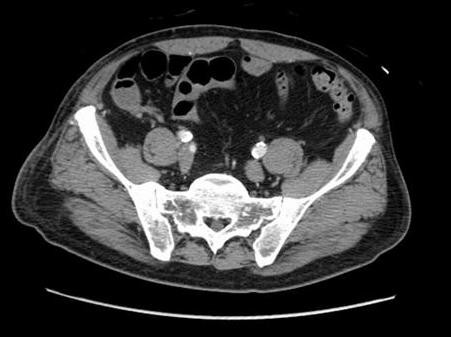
Abdominal CT scan. An angio CT scan showed calcic atheromatous plaques in the abdominal aorta and iliac arteries.
